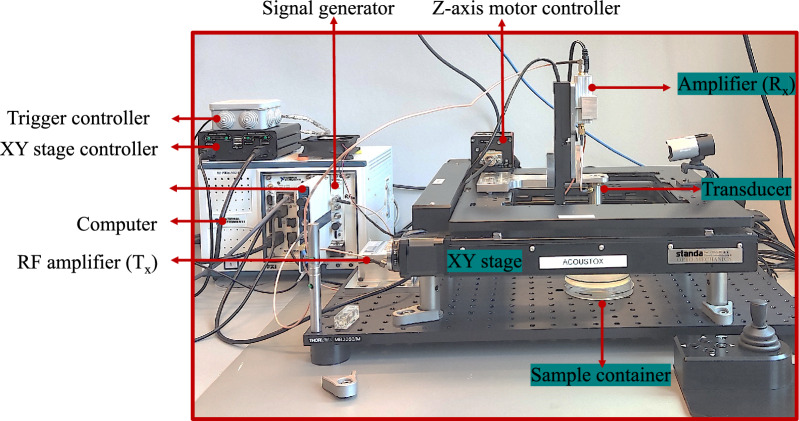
Representation of the SAM setup, which includes a signal generator, RF amplifier ($$T_x$$), and signal receiver amplifier ($$R_x$$) to excite and detect ultrasonic signals. The system is integrated with an XY stage and Z-axis motor controller for precise spatial positioning of the transducer. Automated scanning and data acquisition are managed by a computer-based control unit linked to the trigger and stage controllers. The sample is positioned in a dedicated container to allow controlled ultrasonic wave propagation^55–57^.
